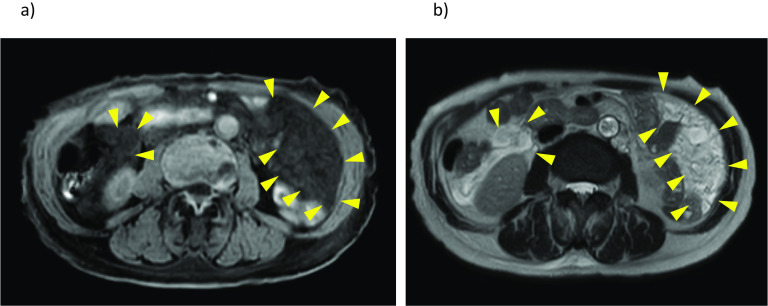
Abdominal magnetic resonance imaging study: Multiple lesions of a T1-weighted and b T2-weighted sequences, showing respective signal intensities (T1, intermediate; T2, high) (arrowhead)
